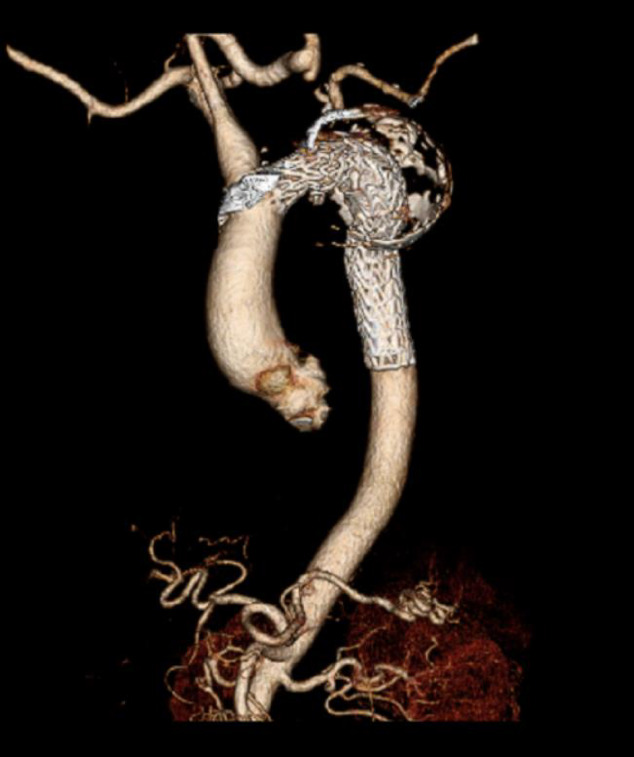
Three-dimensional CTA reconstruction showing repair and exclusion of the aneurysm with TEVAR
